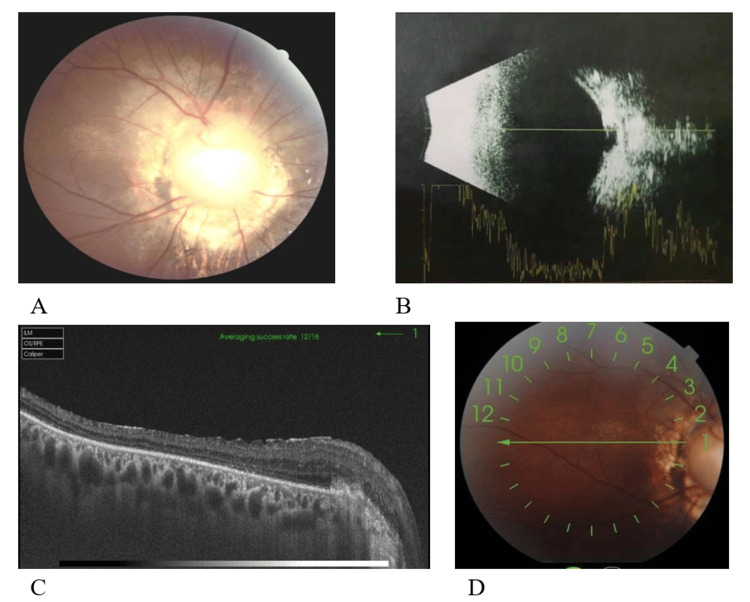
Postoperative ocular examination one month after surgery A: One month post-surgery, fundus photography of the right eye shows the retina has been reattached with laser spots visible around the optic disc. Partial macular structure is preserved, and the best-corrected visual acuity is 2/100. B: B-scan Ultrasonography of the right eye post-surgery: The retina has been reattached, and a depressive change of the optic disc is visible. C & D: OCT of the macular area (circular scan) of the right eye one month post-surgery: The macular area exhibits developmental abnormalities, with no central foveal morphology visible. The retina is atrophic and thinned.
